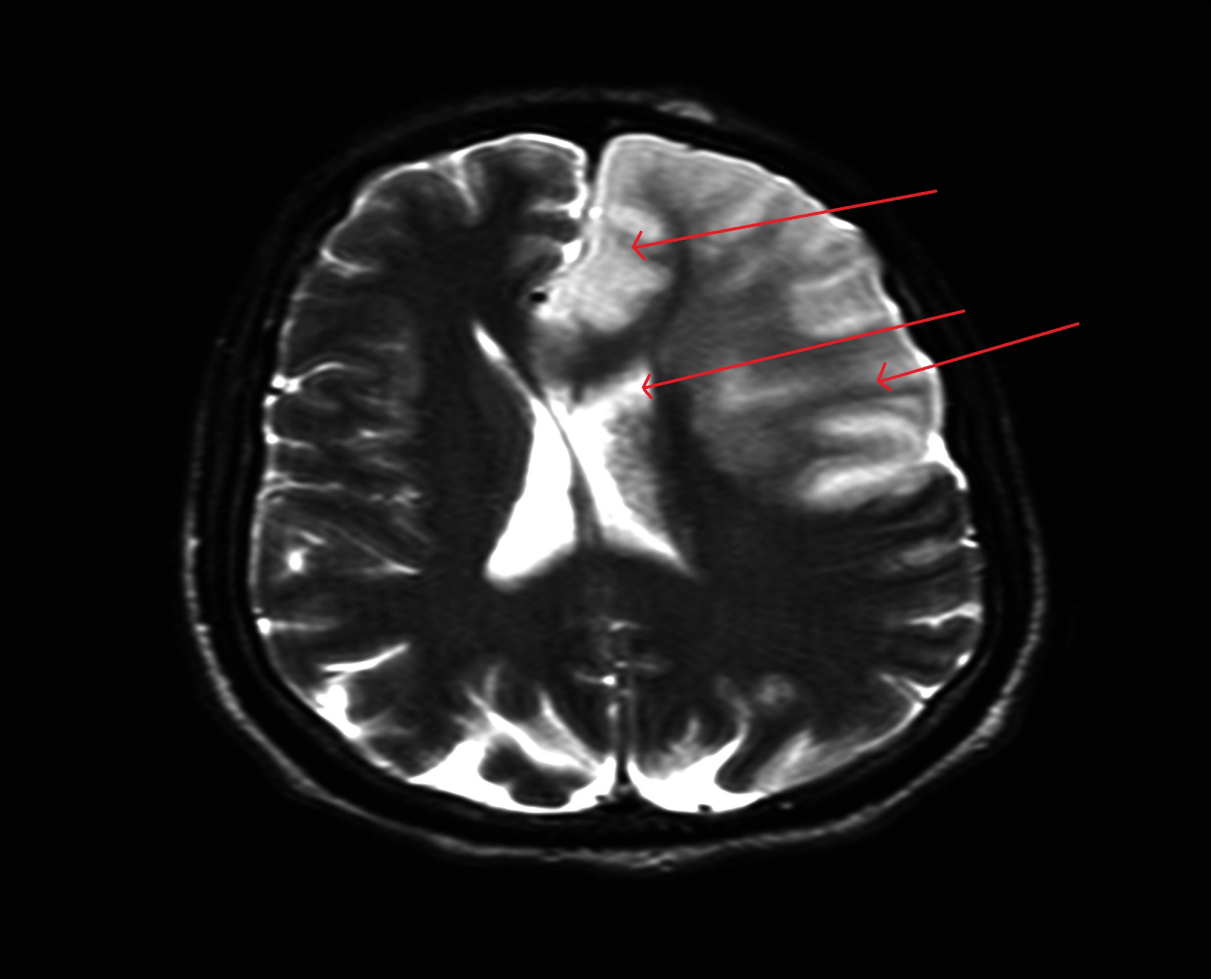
MRI of the brain showing infarction in the left frontal, left parietal, and left caudate area, concerning for cardiac embolization. There is also a midline shift of 5 mm. MRI - Magnetic Resonance Imaging.
